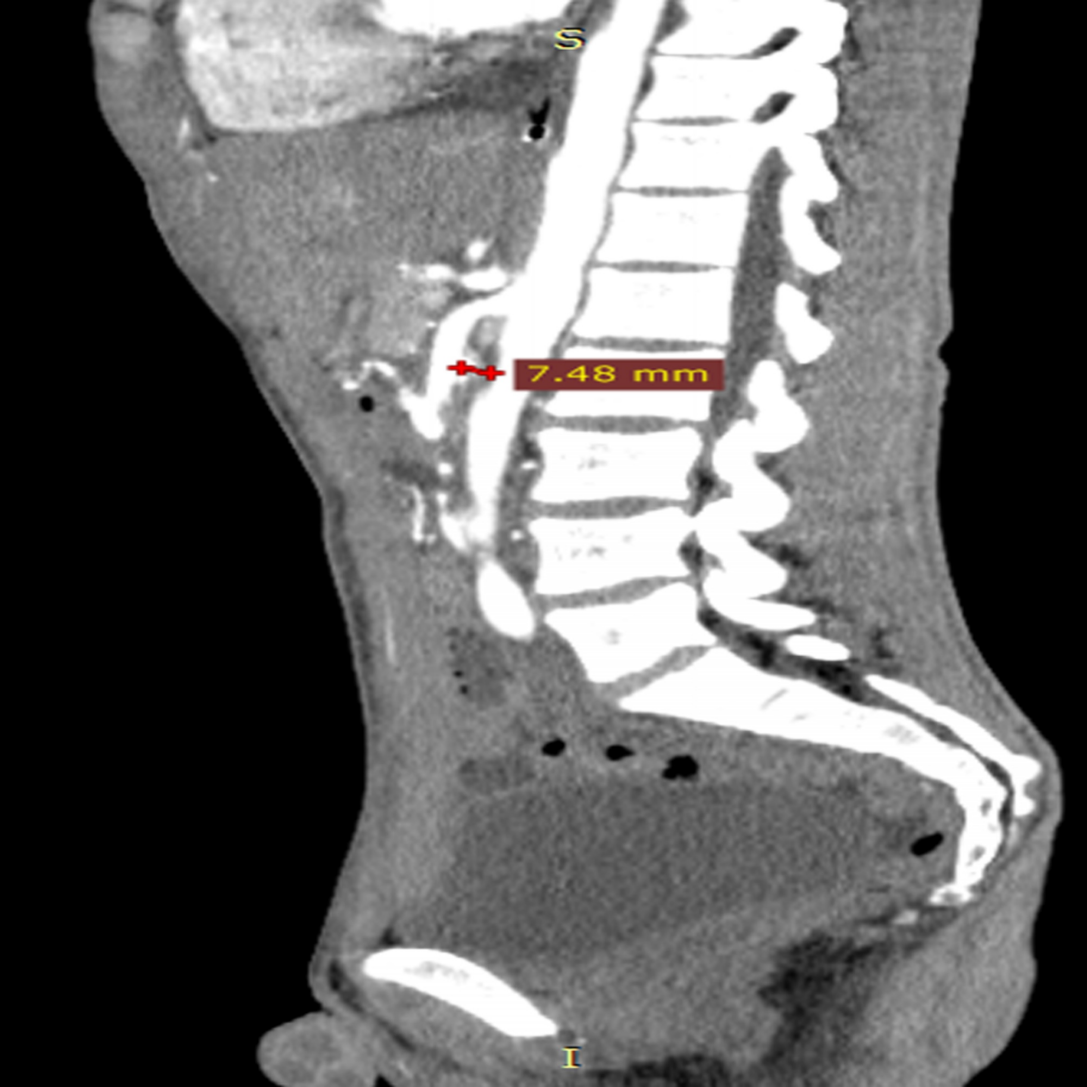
Sagittal computed tomography angiography (CTA) of the abdomen demonstrates a distance of 7.48 mm between the superior mesenteric artery and the aortic artery.
